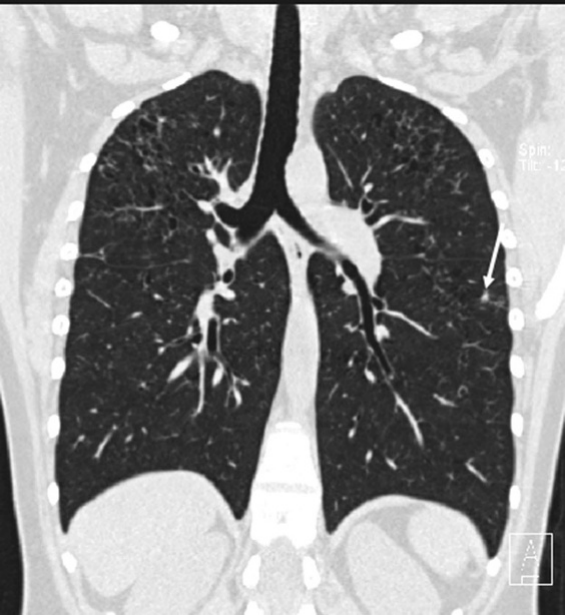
A 41-year-old woman with PLCH. Coronal CT image (thin MIP reconstruction) showing typical distribution of disease: many cysts predominating in upper and middle lungs fiends, with some micronodules (*arrow*); typically, basal regions are spared
